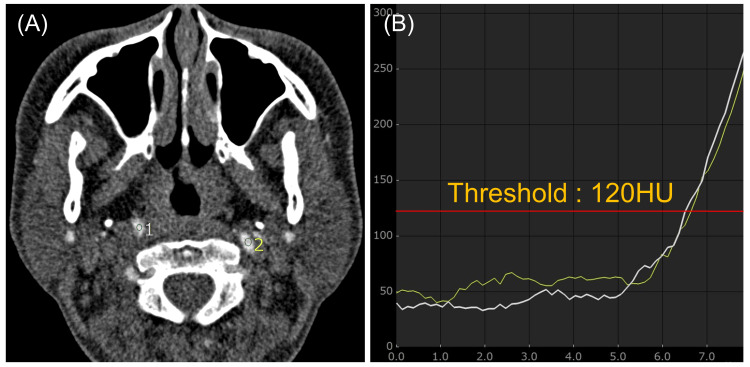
Method of measuring contrast media arrival time A and B: Time enhancement curves were obtained by placing regions of interest on images obtained from computer-aided bolus tracking technology (Real Prep, Canon Medical Systems). Contrast media (CM) arrival time was defined as the time from the start of CM injection until the mean computed tomography (CT) attenuation of the internal carotid artery (ICA) reached 120 Hounsfield units.
